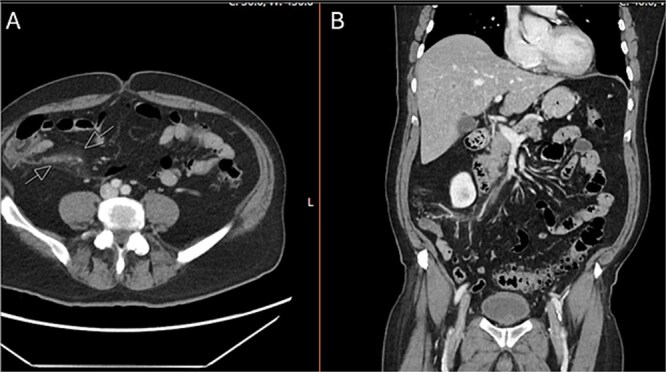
CT abdomen and pelvis with IV contrast. A) Axial and B) coronal series identifying normal appendix and right lower quadrant mesenteric inflammatory changes.
